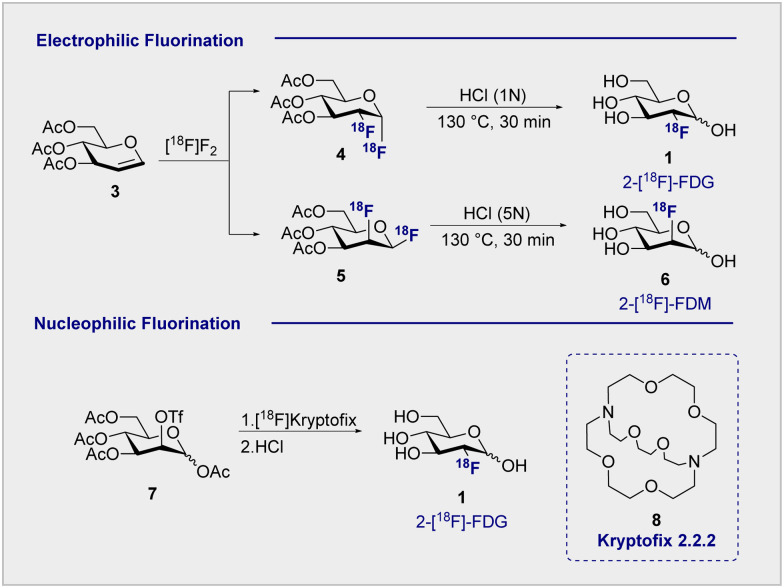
^18^F Fluorination methods: (upper) electrophilic fluorination produces both 2-[^18^F]-FDG (1) and 2-[^18^F]-FDM (6) products; (lower) nucleophilic fluorination produces solely glucose-configured 2-[^18^F]-FDG (1).
